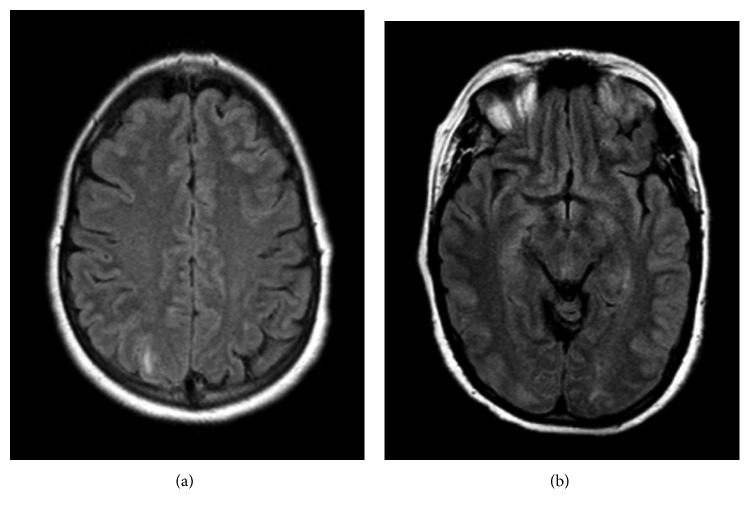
Axial FLAIR images of the brain demonstrating hyperintense signals in the (a) posterior right parietal lobe and (b) posterior occipital lobes bilaterally.
